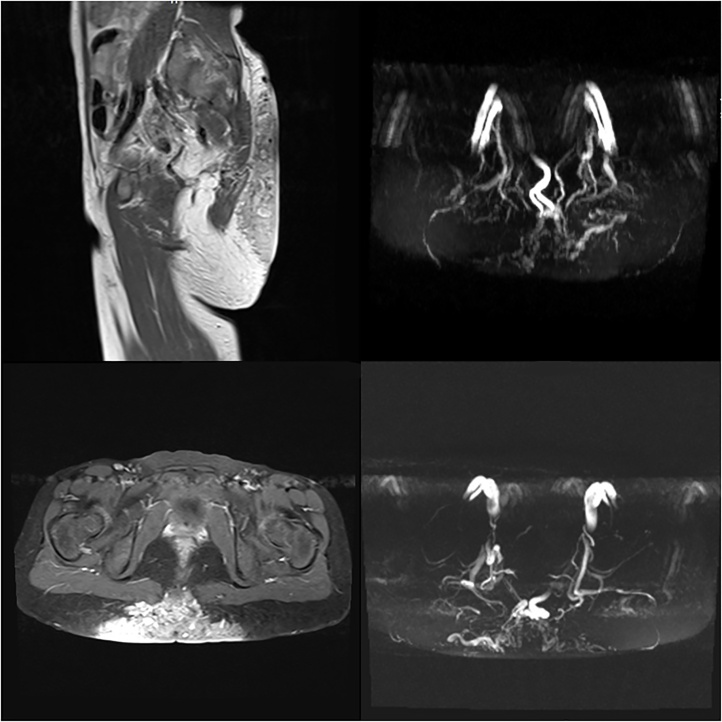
The first MRI of the lesion.
